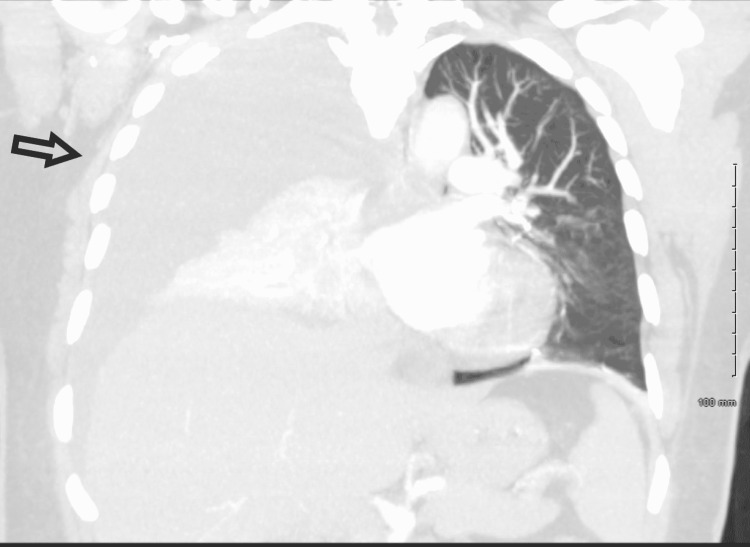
CT chest coronal view showing complete opacification and atelectasis of the right, middle and lower lobe.
